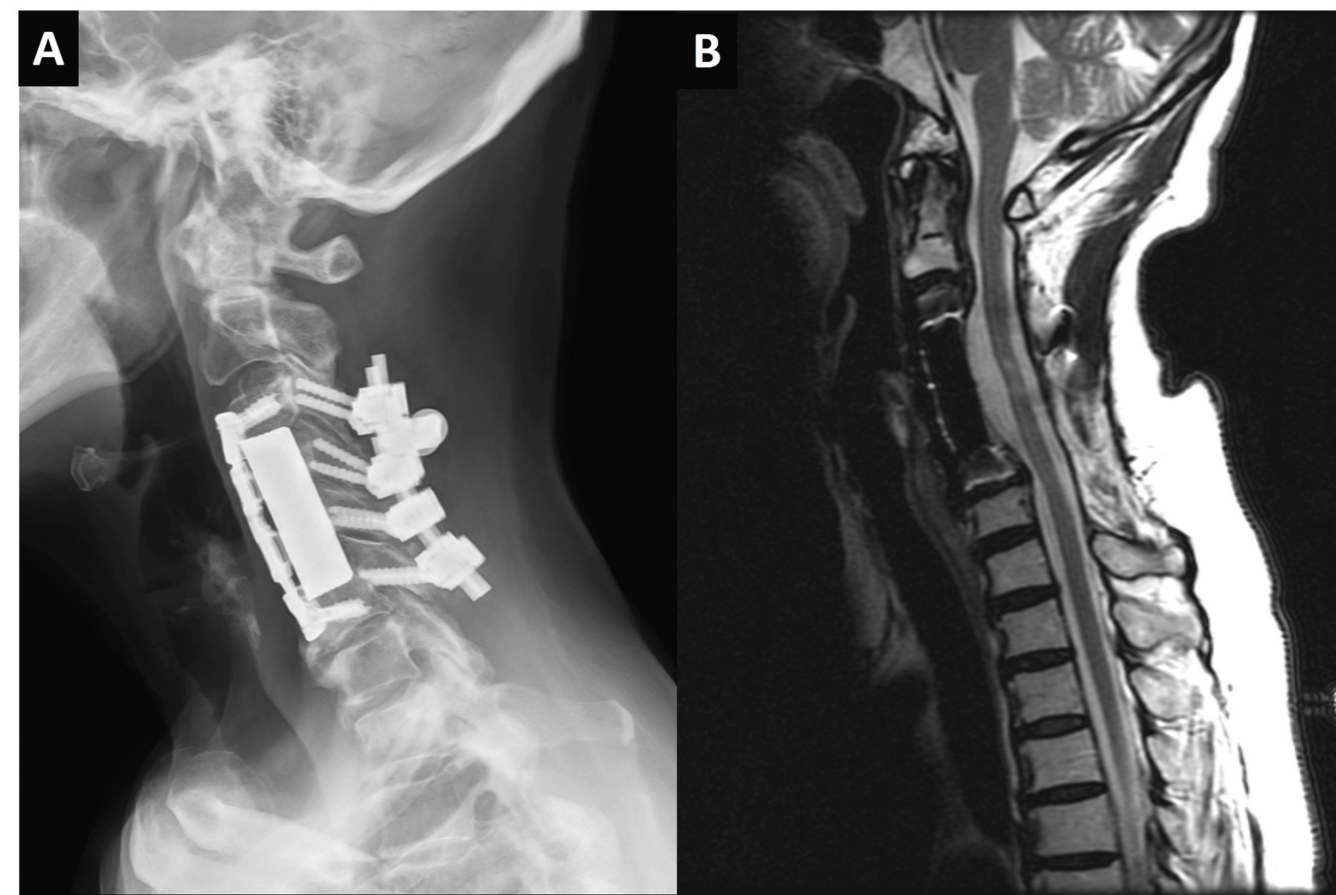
Postoperative follow-up at two years (A) A cervical spine X-ray shows adequate position and alignment of the fusion instruments. (B) A T2-weighted magnetic resonance image reveals satisfactory decompression of the spinal cord without recurrence of stenosis.
